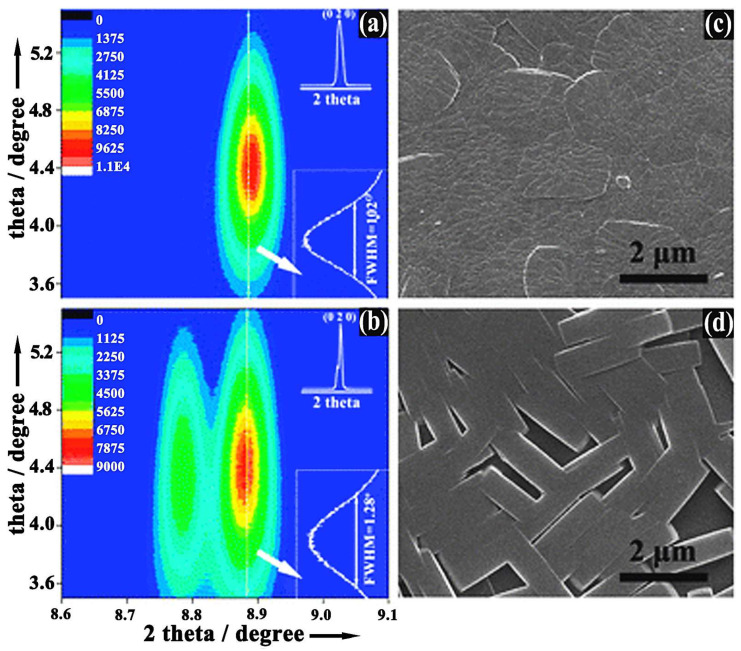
Comparison of *b*-oriented MFI zeolite films prepared by (a,c) microwave and (b,d) conventional synthesis: (a,b) X-ray rocking curve analysis; (c,d) SEM images of membrane surfaces. Redrawn from Ref. [92]. Copyright 2012, Royal Society of Chemistry.
